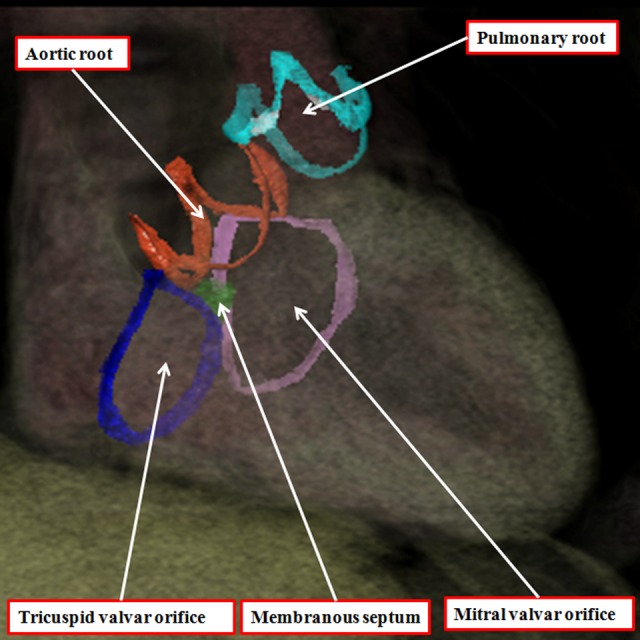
The three-dimensional data set made available by means of multidetector computed tomography from a patient undergoing investigation of coronary arterial disease has been segmented and reconstructed to show the hinges of the leaflets of the arterial and atrioventricular valves. The leaflets of the atrioventricular valves are hinged in an annular fashion, whereas the hinges of the arterial valves, when reconstructed, take the form of three-pointed coronets.
